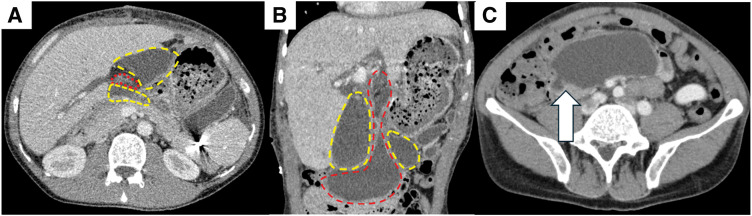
CT image on POD 16. (A) Abscess area surrounding the cholangiojejunostomy was observed by axial view. (B) Coronal view of the image. (C) Beak sign and dilatation of the proximal bowel by axial view. Red dot area: Dilated afferent jejunal loop, Yellow dot area: Abscess, White arrow: Beak sign.
